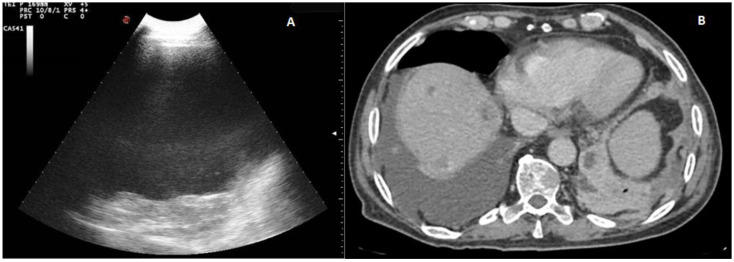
(A) TUS scan showing a homogeneous iperechoic pleural exudates viewed by a convex multifrequency probe (3.5 MHz). The drained fluid was macroscopically hemorrhagic. (B) The corresponding CT scan shows a large right effusion in a patient with a diagnosis of metastatic kidney cancer.
